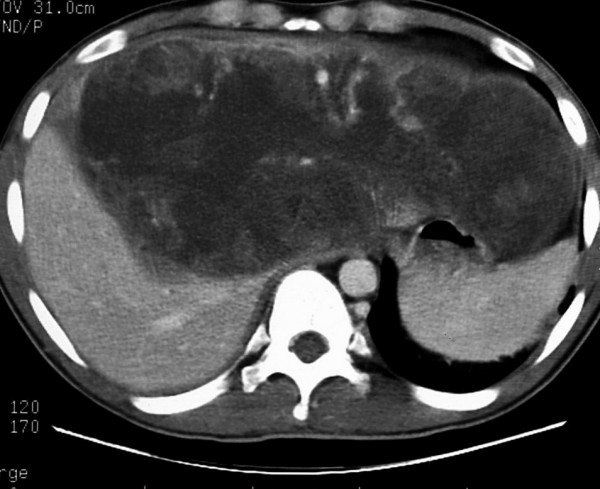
Computed tomography (CT) of the abdomen demonstrating hepatomegaly and a large hypodense lesion of the liver with multicystic appearance with septations and solid portions.
